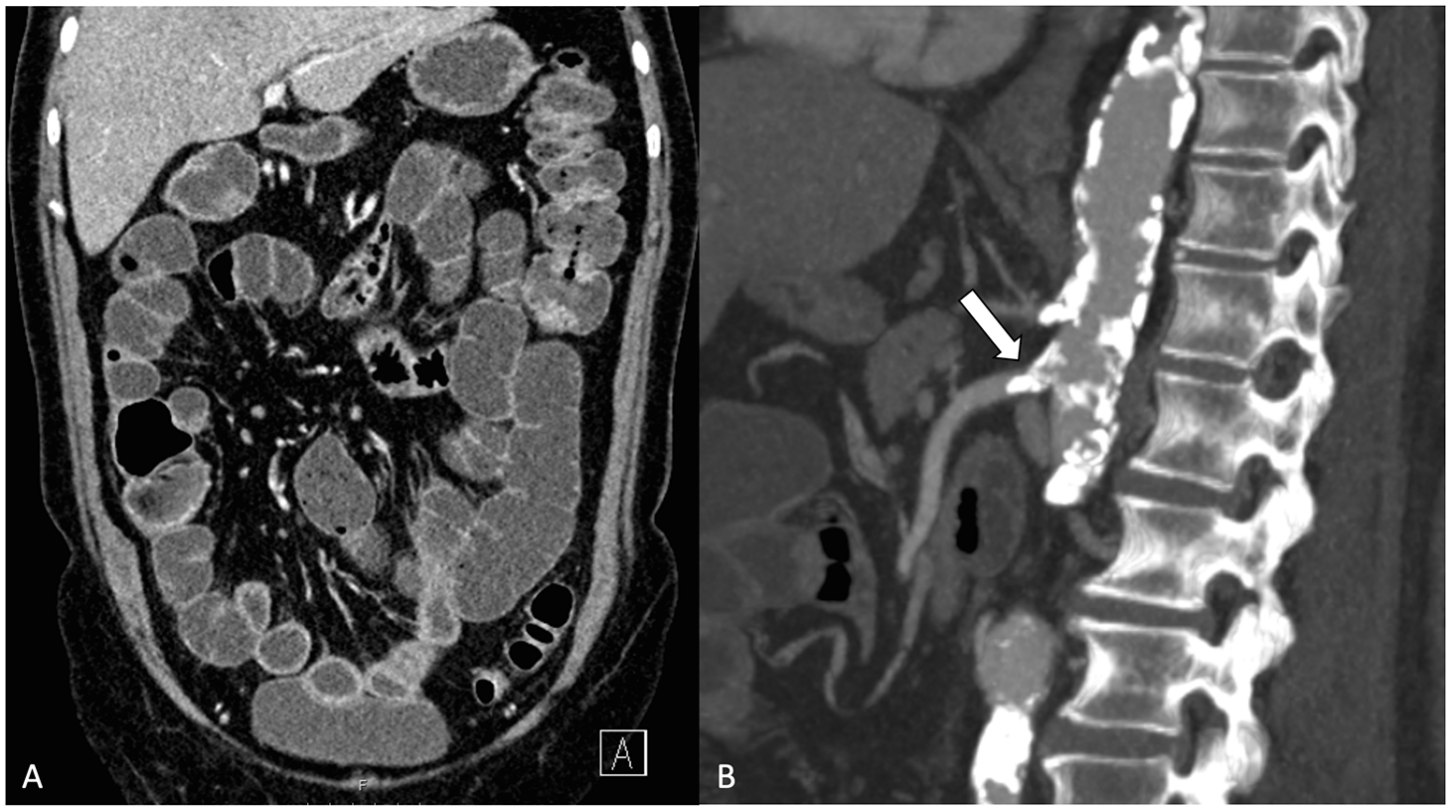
This 70-year-old patient had suffered from postprandial pain and diarrhea for 2 months prior to the final admission. The symptoms worsened and became persistent, and the patient was admitted to the emergency room. C-reactive protein was 400 mg/L. A) Computed tomography showed dilated small bowel loops, bowel wall thickening, mesenteric fat stranding, and abnormal increased enhancement of the intestine. B) All mesenteric arteries were severely obstructed by atherosclerotic disease. Based on the vascular and intestinal findings in the imaging, appropriate clinical presentation, and absence of a competing cause, the diagnosis of acute-on-chronic mesenteric ischemia was made. An exploratory laparotomy was performed mainly due to the high inflammatory markers and clinical suspicion of bowel necrosis. The small bowel appeared pale and cyanotic. However, there was no irreversible bowel damage, and subsequent endovascular stenting of the proximal superior mesenteric artery stenosis (arrow) was performed. The patient recovered without need for bowel resection.
